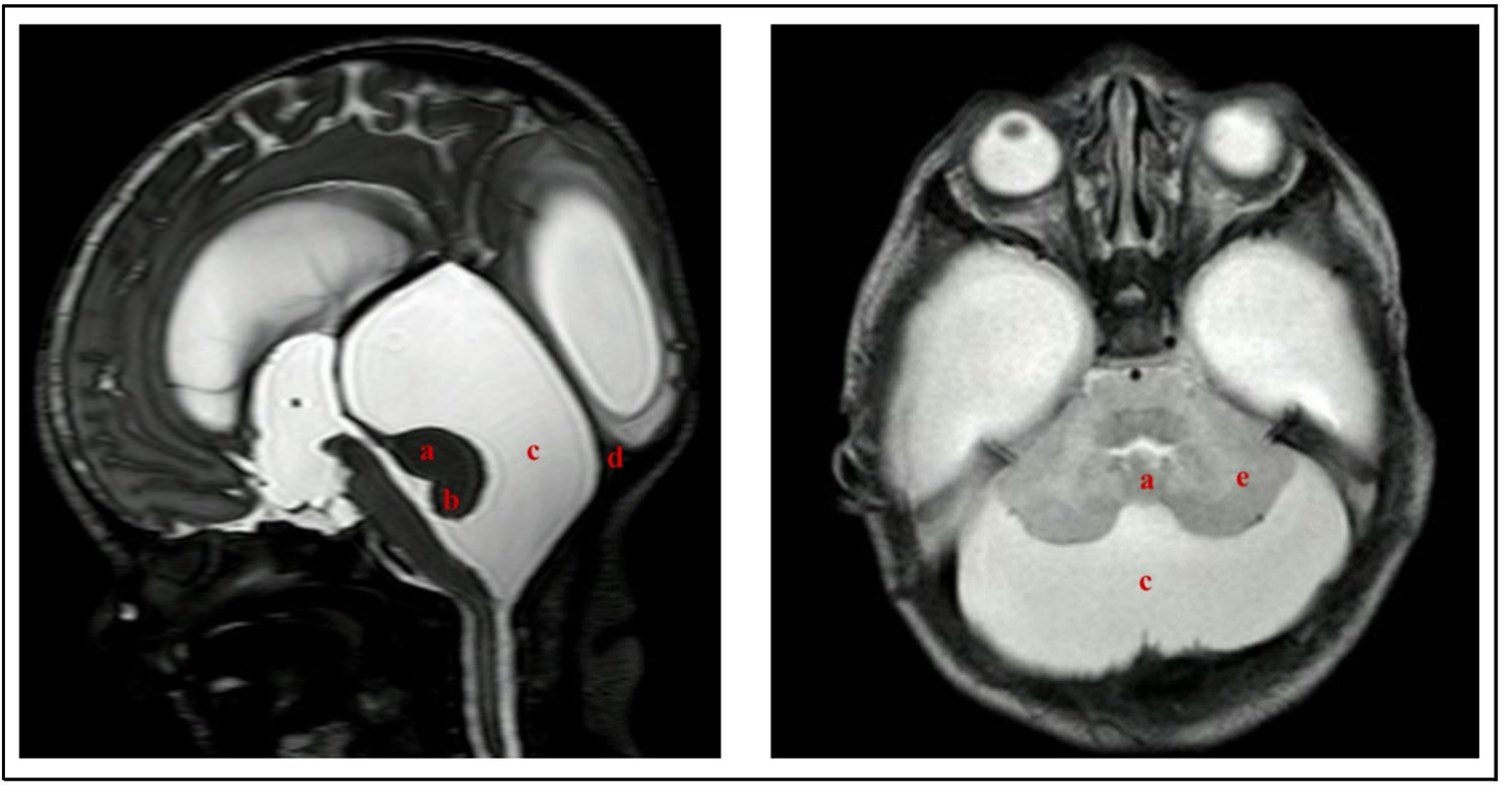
Postnatal sagittal (left) and axial (right) T2-weighted MRI of Dandy-Walker malformation. (a) Hypoplasia, anterior rotation, and superior displacement of the remaining portion of the vermis. (b) Agenesis of the inferior portion of the vermis. (c) Large posterior fossa cyst communicating with the fourth ventricle. (d) Upward displacement of the tentorium and torcula. (e) Anterolateral displacement of the cerebellar hemispheres. Note the large supratentorial ventricular dilation characteristic of hydrocephalus
